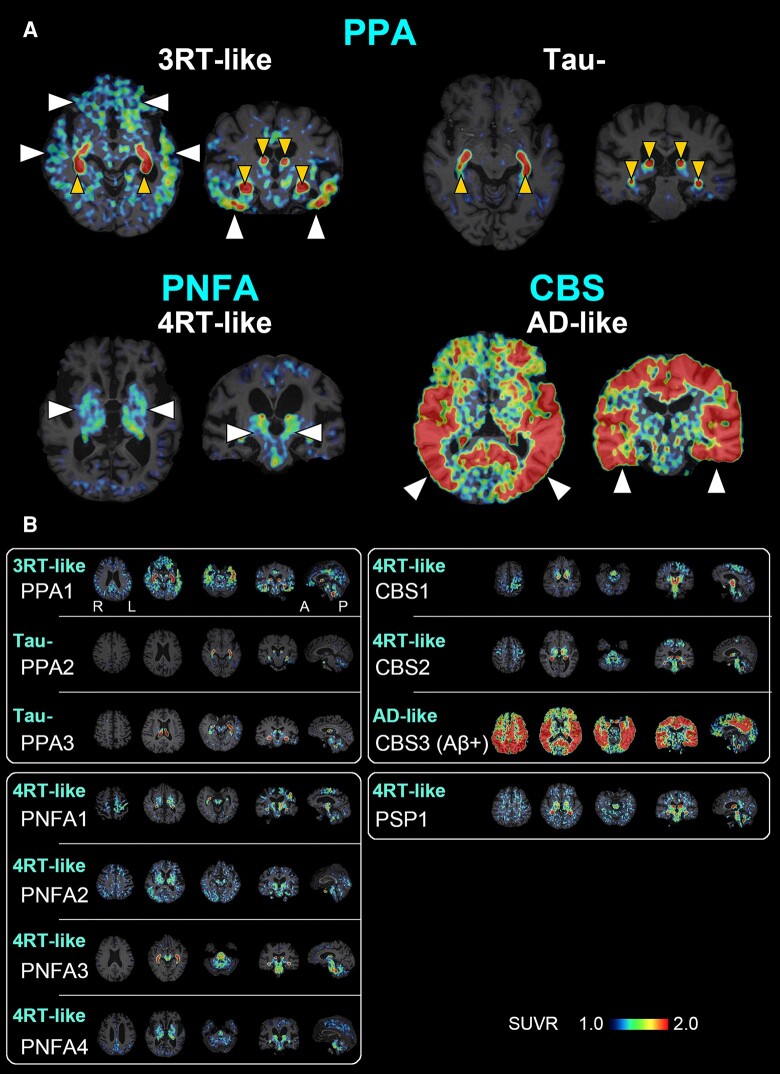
A PET-based subtyping of PPA, PNFA, CBS and PSP. (A) Representative axial (left) and coronal (right) images of radioactivity SUVRs at 90–110 min after ^18^F-florzolotau administration. The arrowheads of normal size indicate enhanced parenchymal radioligand retentions characteristic of each putative tau topology subtype. The smaller arrowheads denote radioactivity accumulations in the choroid plexus supposedly unrelated to tau depositions. Radio signal intensification primarily in the frontotemporal regions and less involvement of posterior and subcortical areas are suggestive of Pick’s disease–type 3RT aggregations in a case with PPA (PPA1), while negativity for tau depositions is indicated in another case with PPA (PPA2). Radio signal increases predominantly in subcortical regions implying PSP- or CBD-type 4RT deposits in a case with PNFA (PNFA4). A case with Aβ-PET–positive CBS (CBS3) exhibited widespread and highly intensified limbic and neocortical radioligand accumulations involving temporo-parietal regions, which is indicative of CBS due to Alzheimer’s disease pathologies. (B) The characterization of putative brain pathologies in three PPA (PPA1–3), four PNFA (PNFA1–4), three CBS (CBS1–3) and one PSP (PSP1) patients by the visual read of tau PET images. Cases with PPA were characterized as 3RT-like (PPA1) and tau-negative (PPA2 and 3), whereas all cases with PNFA were categorized as 4RT-like. Image findings in cases with CBS were either 4RT-like (CBS1, 2) or Alzheimer’s disease–like (CBS3), and radio signal distribution in the PSP-FTD case was consistent with 4RT-like pathology. In each subject, three axial, one coronal and one sagittal section providing characteristic information are presented from the left. A, anterior; L, left, P, posterior; R, right.
